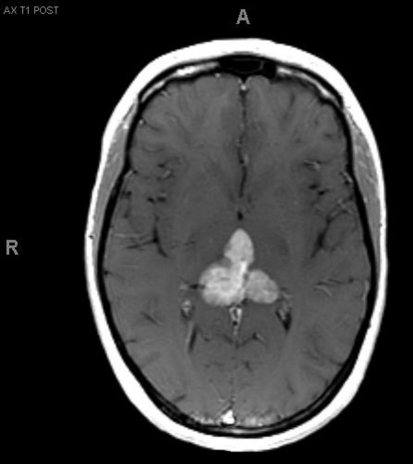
MRI after first resection.
